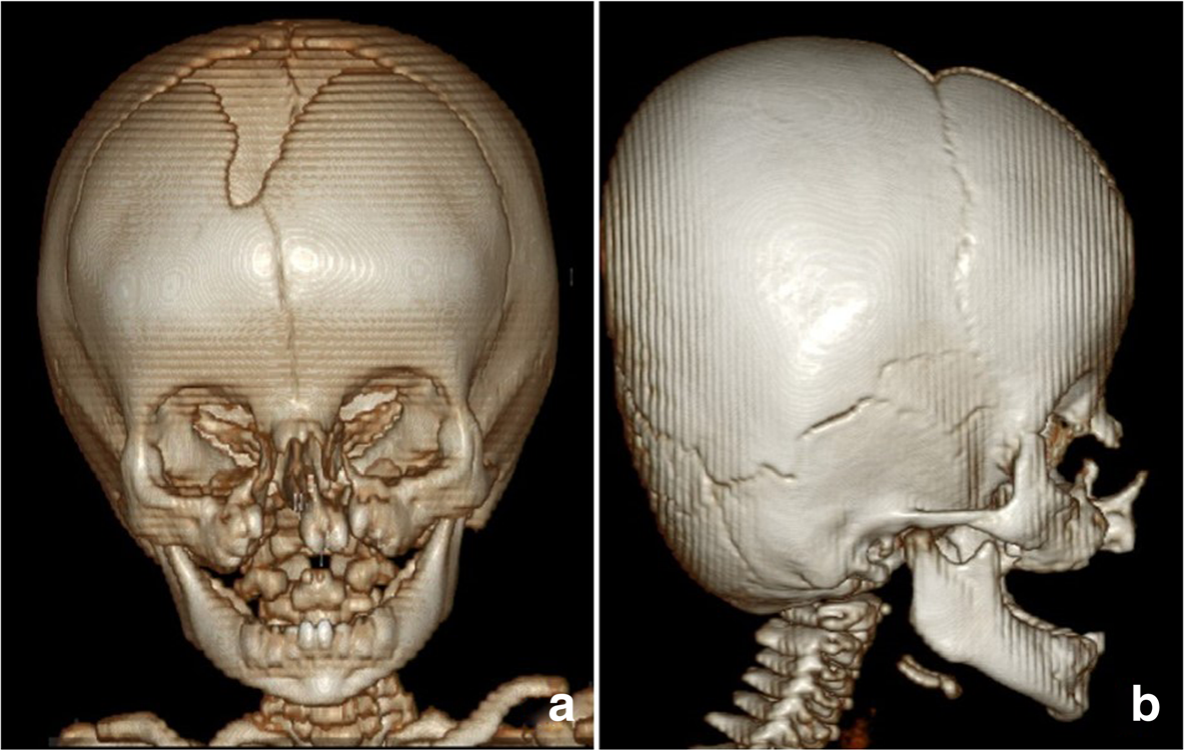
3D CT views. a The frontal view demonstrated corresponding bony clefts extended from the alveolus, through the nasomaxillary sutures, and up to the inferomedial walls of the orbits. b The lateral view demonstrated a protruding premaxilla
